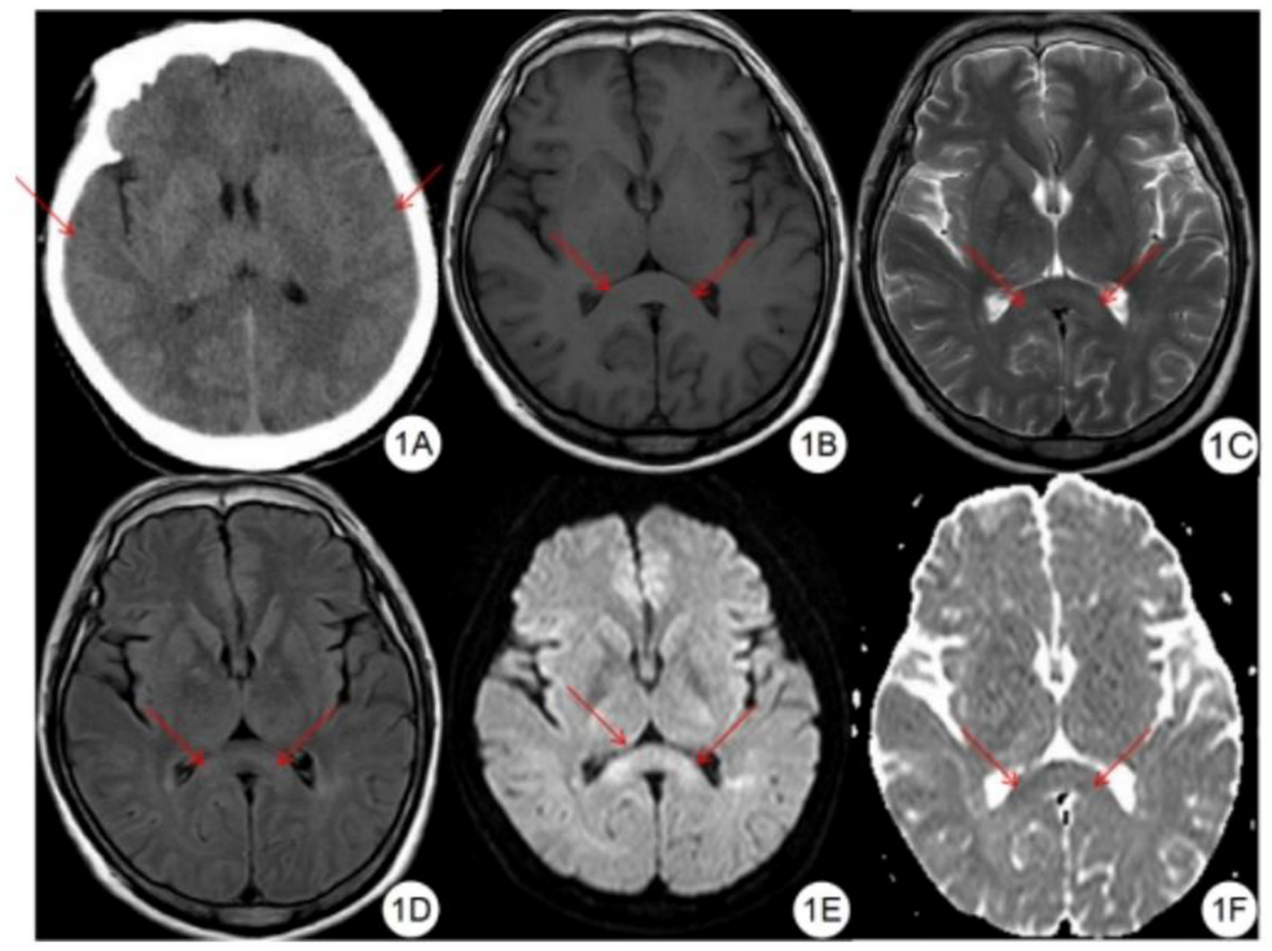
Mild HACE patient. (A) CT plain scan showed that the sulcus and cerebral fissure were slightly shallower. (B) T1WI showed symmetrical slightly hypointensity in the splenium of the corpus callosum. (C) T2WI showed symmetrical slightly hyperintensity in the splenium of the corpus callosum. (D) FLAIR showed symmetrical slightly hyperintensity in the splenium of the corpus callosum. (E) DWI showed symmetrical hyperintensity in the splenium of the corpus callosum. (F) ADC shows symmetrical hypointensity in the splenium of the corpus callosum.
